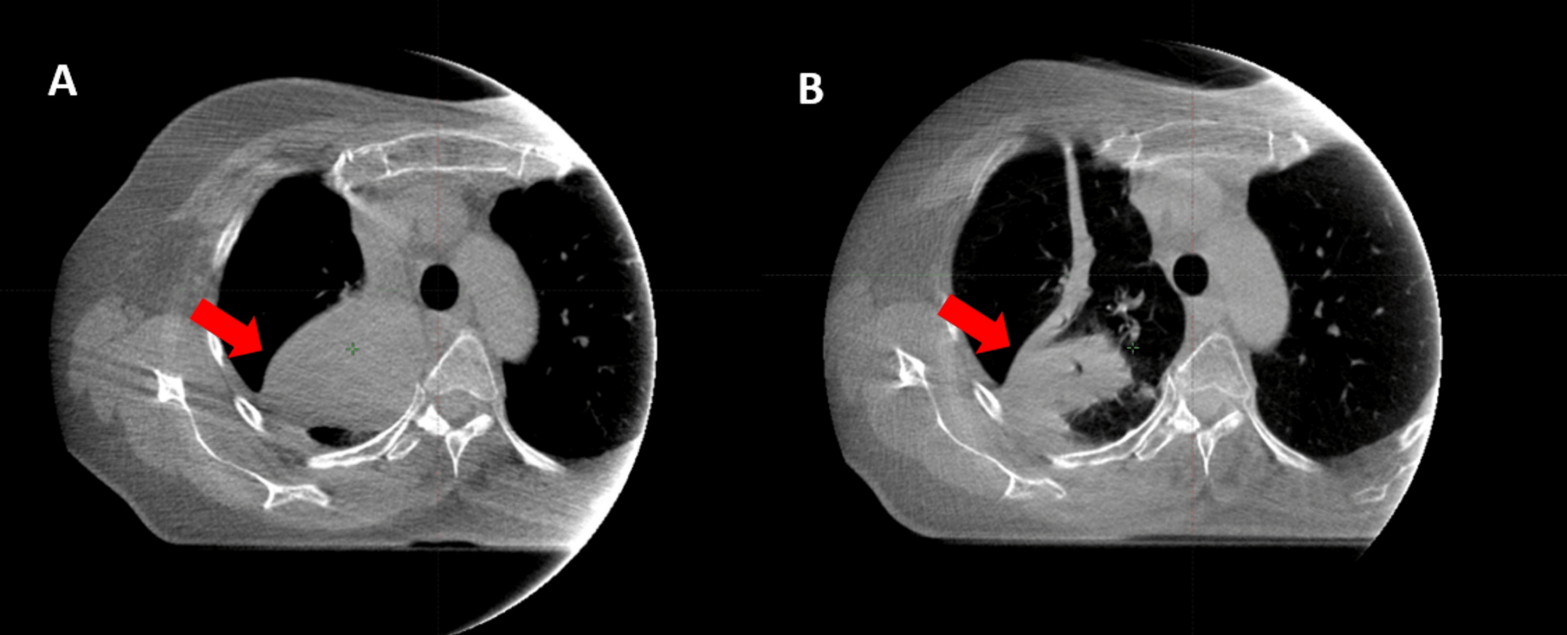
Comparison of tumor size and shape (indicated by the red arrow) on the first CBCT (A) and the 21st CBCT (B) CBCT: Cone-beam computed tomography
